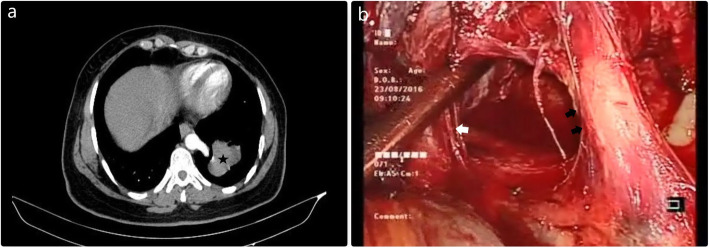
Extralobar sequestration (Asterisk) was seen in the left lower lobe, and the arterial supply of the pulmonary sequestration was detected preoperatively via the thorax CT (Fig. 3a). VATS resection was performed. Arterial blood supply (Black arrows) from the aorta was seen intraoperatively and dissected with an endoscopic vascular stapler (Fig. 3b). The white arrow indicates the pulmonary vein
